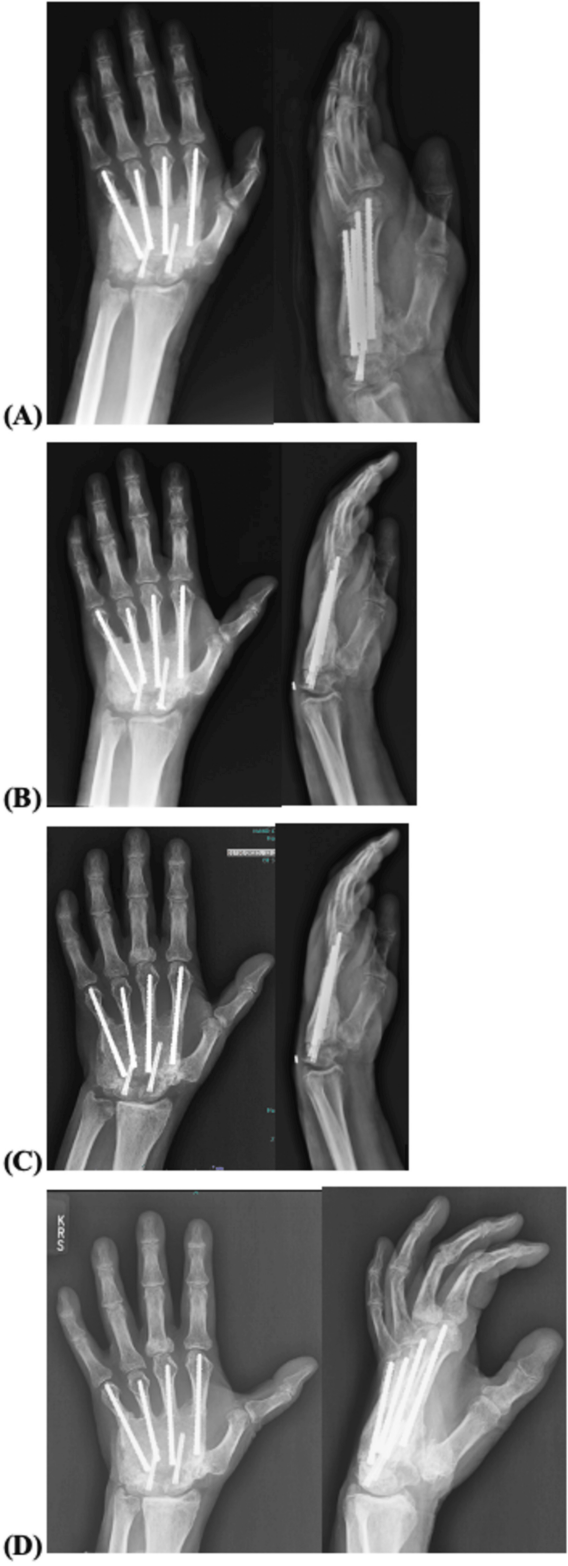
Anterior-posterior and lateral views of left-hand radiographs. Radiographs at (A) one month, (B) three months, (C) six months, and (D) one year after reconstructive surgery showing stable fusion of the graft.
